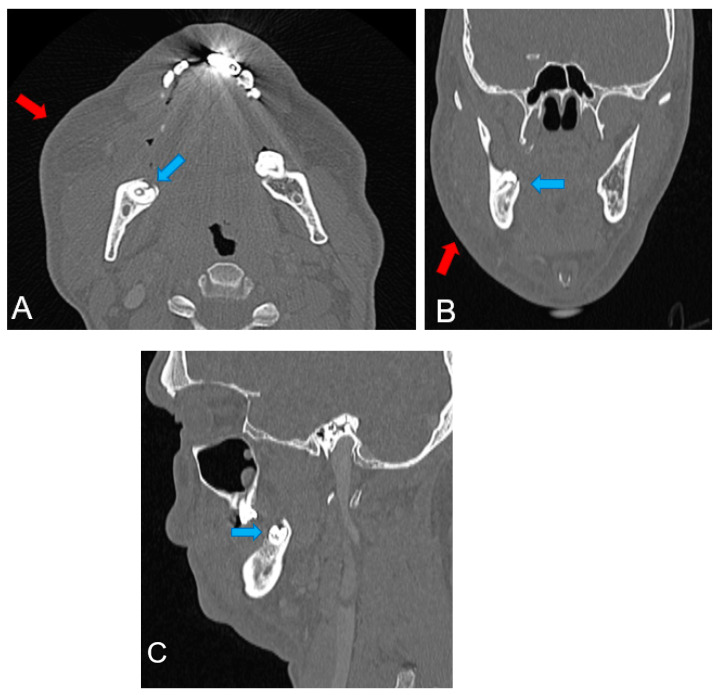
CT scan examination: (A) axial view; (B) frontal view; (C) sagittal view. The red arrow in (A,B) indicates the facial cellulitis lesion and the blue arrow in (A–C) the coronal fracture of the impacted lower right wisdom tooth.
